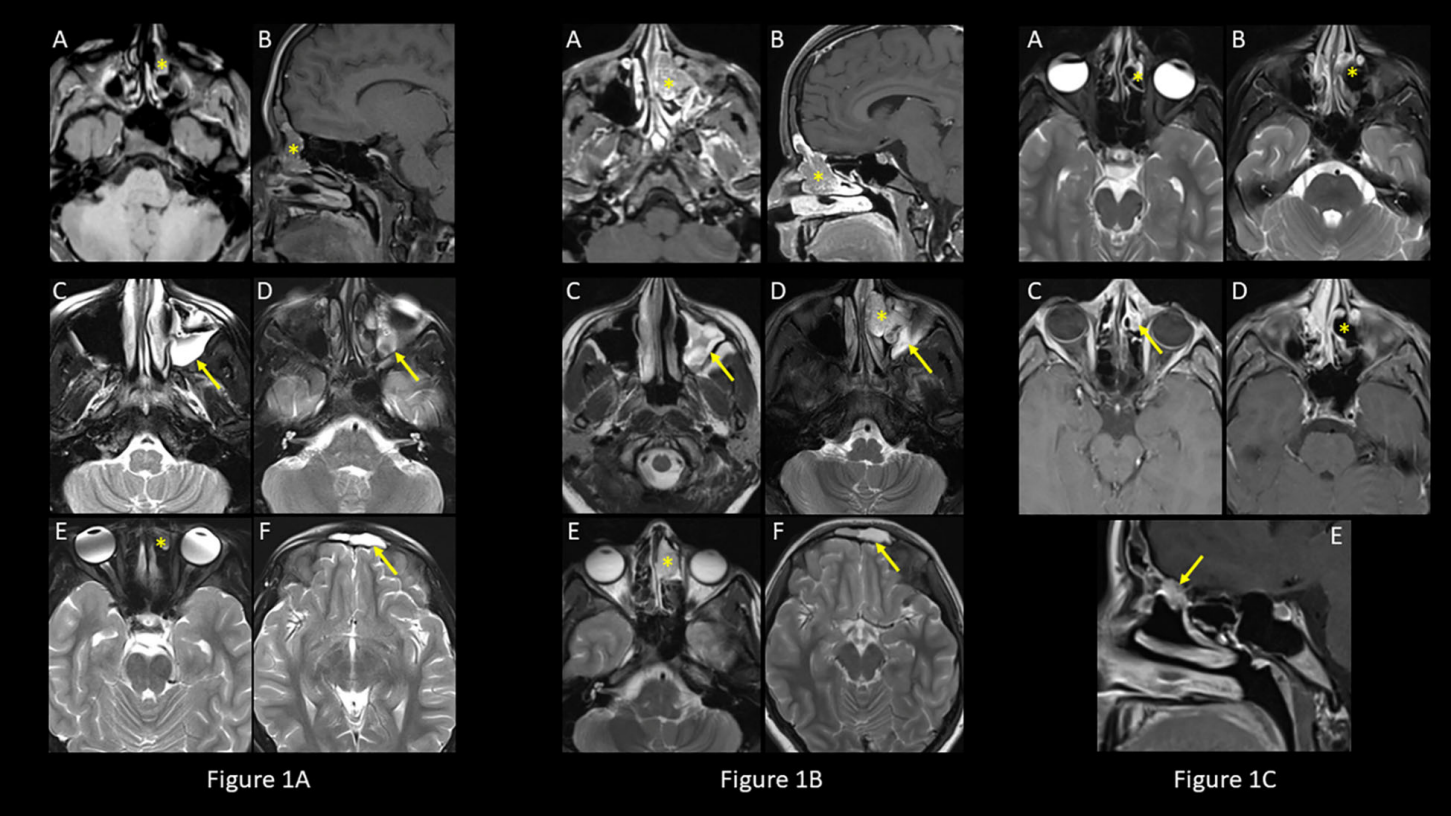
Initial and Follow-up MRI findings. (A) Initial MRI exam. Axial (A) and sagittal (B) post-contrast T1-weighted and axial T2-weighted (E) images demonstrate nonspecific enhancing material in left frontoethmoidal sinus (asterisk) with fluid and mucoperiosteal thickening in the maxillary and frontal sinuses on axial T2-weighted images (C, D, F; arrows). (B) Follow-up MRI exam, 31 months after the initial MRI exam. Lobulated contrast enhancing mass on axial (A) and sagittal (B) post-contrast T1-weighted and axial T2-weighted (D, E) images (asterisk) with mucoperiosteal thickening and fluid in the maxillary and frontal sinuses on axial T2-weighted images (C, D, F; arrows). (C) Post operative MRI exam. Naso-ethmoid resection site (asterisks) demonstrated on axial T2-weighted (A, B) and post-contrast T1-weighted (D) images, with minimal residual enhancing soft tissue (yellow arrow) in the roof of the nasal fossa at the fovea ethmoidalis on axial (C) and sagittal (E) post-contrast T1-weighted images (arrow) consistent with persistent tumor remnant in keeping with positive surgical margins.
